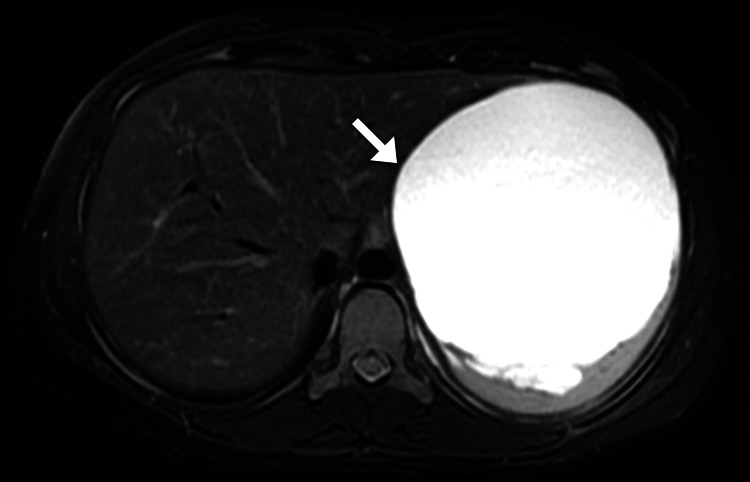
Axial T2-weighted MRI of the abdomen, displaying a lesion with high signal intensity (arrow) within the spleen. MRI: magnetic resonance imaging
